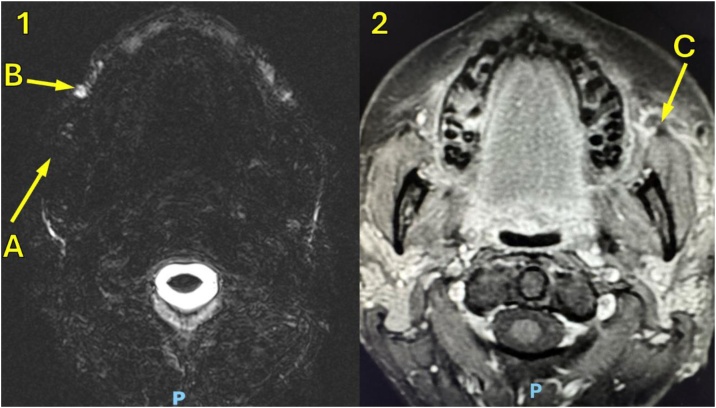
(1) Axial MRI, T2, with filling material (Silicone) occluding the parotid duct by compression. (A) Duct filling failure. (B) Filling material (Silicone). (2) Axial MRI, T1with partial parotid duct lesion in the anterior border of masseter muscle and salivary fistula. (C) Partial Parotid Duct lesion with salivary fistula.
